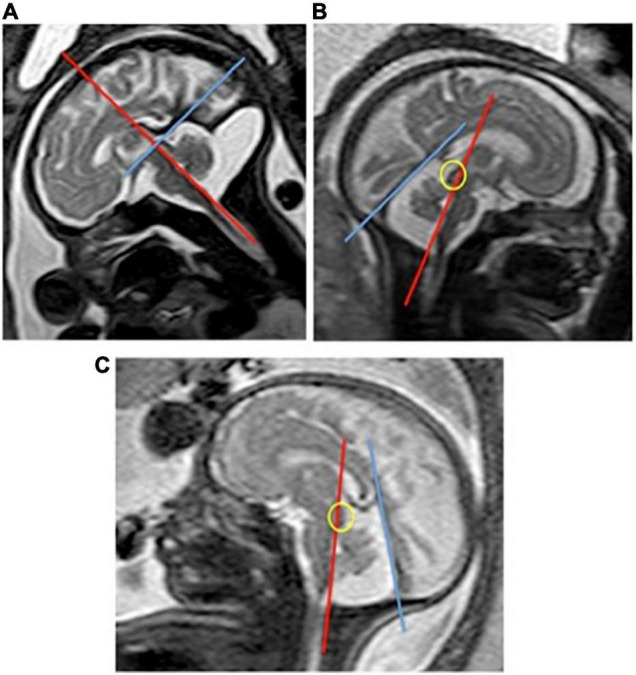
Fetal MRI showing difficulties in the measurement of the BT angle. (A) Shows a fetal MRI where the tentorial surface is irregular due to the PFFC. The line that goes from the quadrigeminal plate and follows the tentorium does not represent the tentorial insertion in the occipital bone. (B,C) Show two examples of fetuses where it is not possible to draw a line that would go from the quadrigeminal plate (circle) following the tentorial surface.
